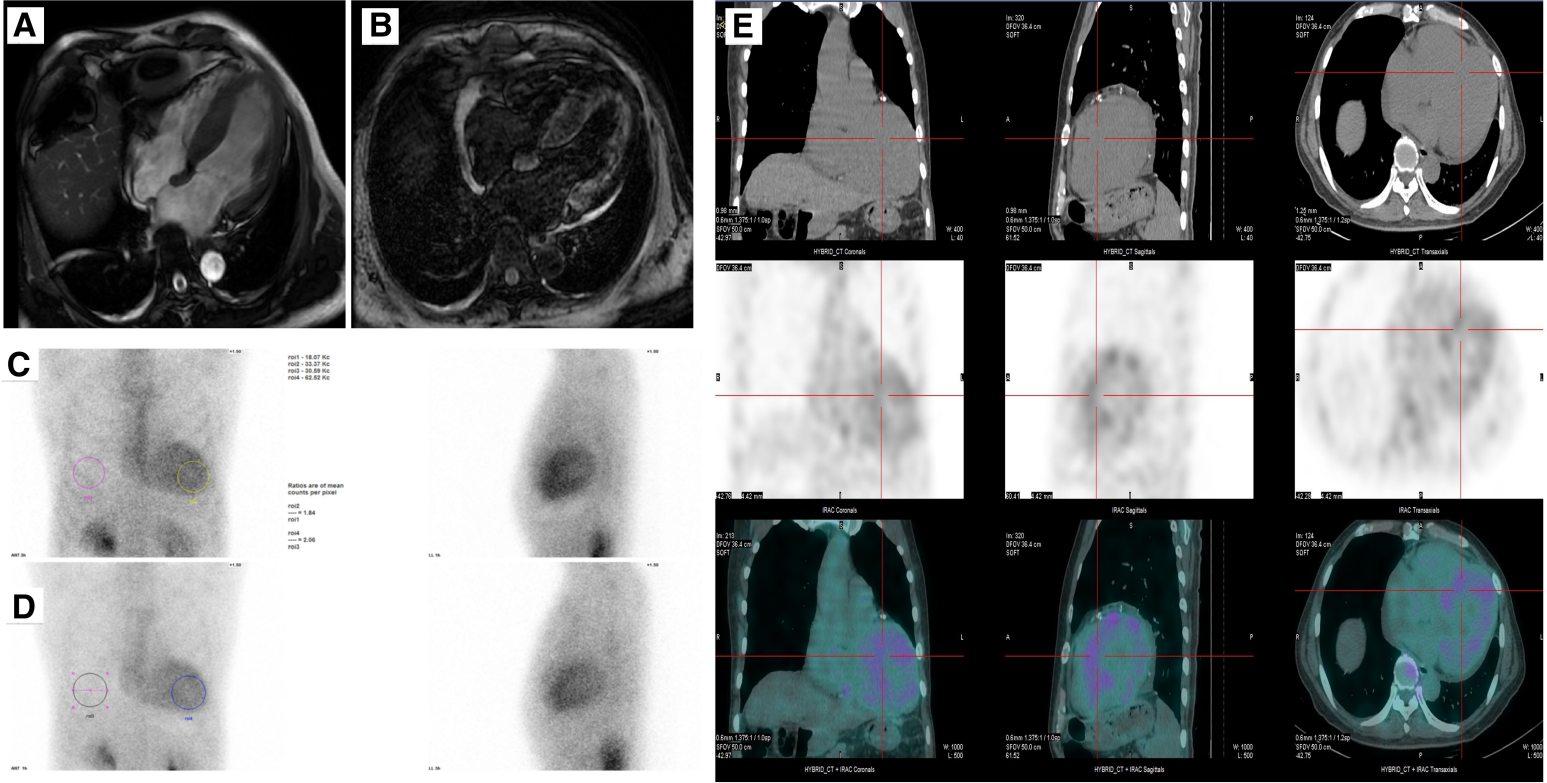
(A,B) Cine and late gadolinium-enhanced MRI. (C–E) A SPECT imaging using 99mTc-PYP scintigraphy showing uptake of 99mTc-PYP by cardiac at 3 h and 1 h.
